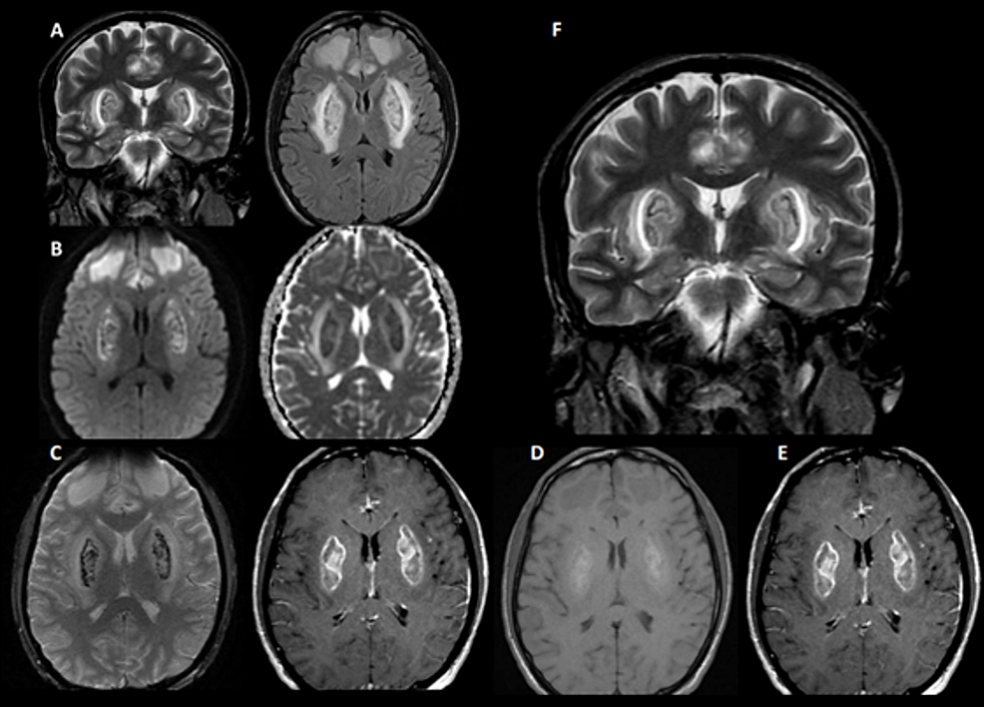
MRI with bilateral and symmetrical basal ganglia lesions. A) Areas of hyperintensity in T2 and T2 FLAIR deep white substance of the frontal lobes, anterior portions of the cingulate/medial region of the frontal lobes, and striated bodies. B) Restriction to the diffusion of water in DWI (hypersignal in DWI and hyposignal in ADC) symmetrically affecting the deep white substance of the frontal lobes, anterior portions of the cingulate/medial region of the frontal lobes, and corpus striatum. C) With the exception of the deep white matter of the frontal lobes, the other lesions had foci of low signal on T2* and high signal on T1, suggesting hemorrhage. D) T1 pre-contrast image. E) T1 post-contrast image showing predominantly peripheral enhancement by contrast around the lesions in the basal ganglia, bilaterally. Leptomeningeal enhancement was observed adjacent to the cingulate gyri and the medial regions of the frontal lobes. F) Visualization of the coronal T2 with hypersignals affecting the symmetrical corpus striatum. T1: T1-weighted pulse sequence; T2: T2-weighted pulse sequence; T2*: echo gradient weighting; FLAIR, fluid-attenuated inversion recovery; DWI: diffusion-weighted imaging; ADC: apparent diffusion coefficient
